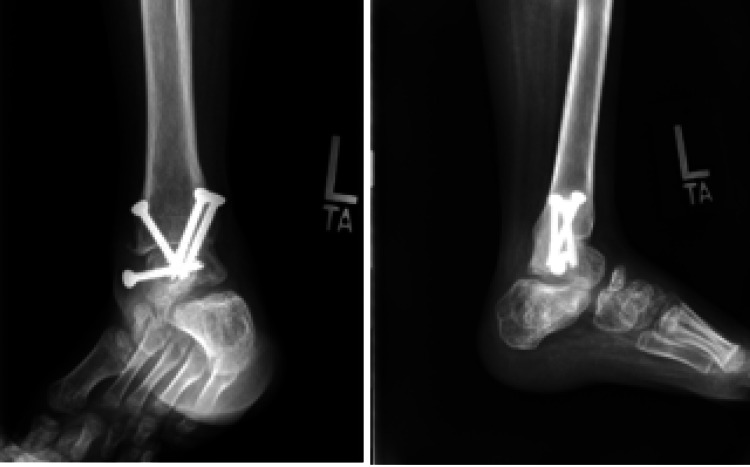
Follow-up radiography Anteroposterior (Panel A) and lateral (Panel B) radiographs of the left ankle 14 months after the procedure demonstrate a consolidated tibiotalar arthrodesis.
